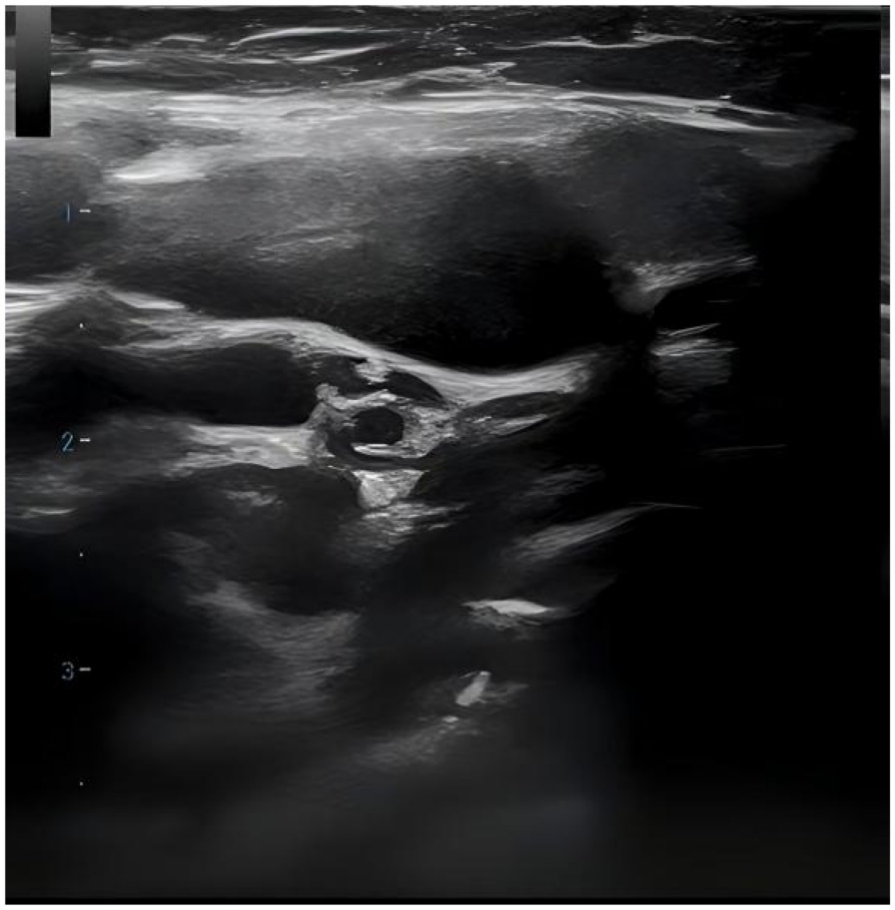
Ultrasonogram of lymph node.
